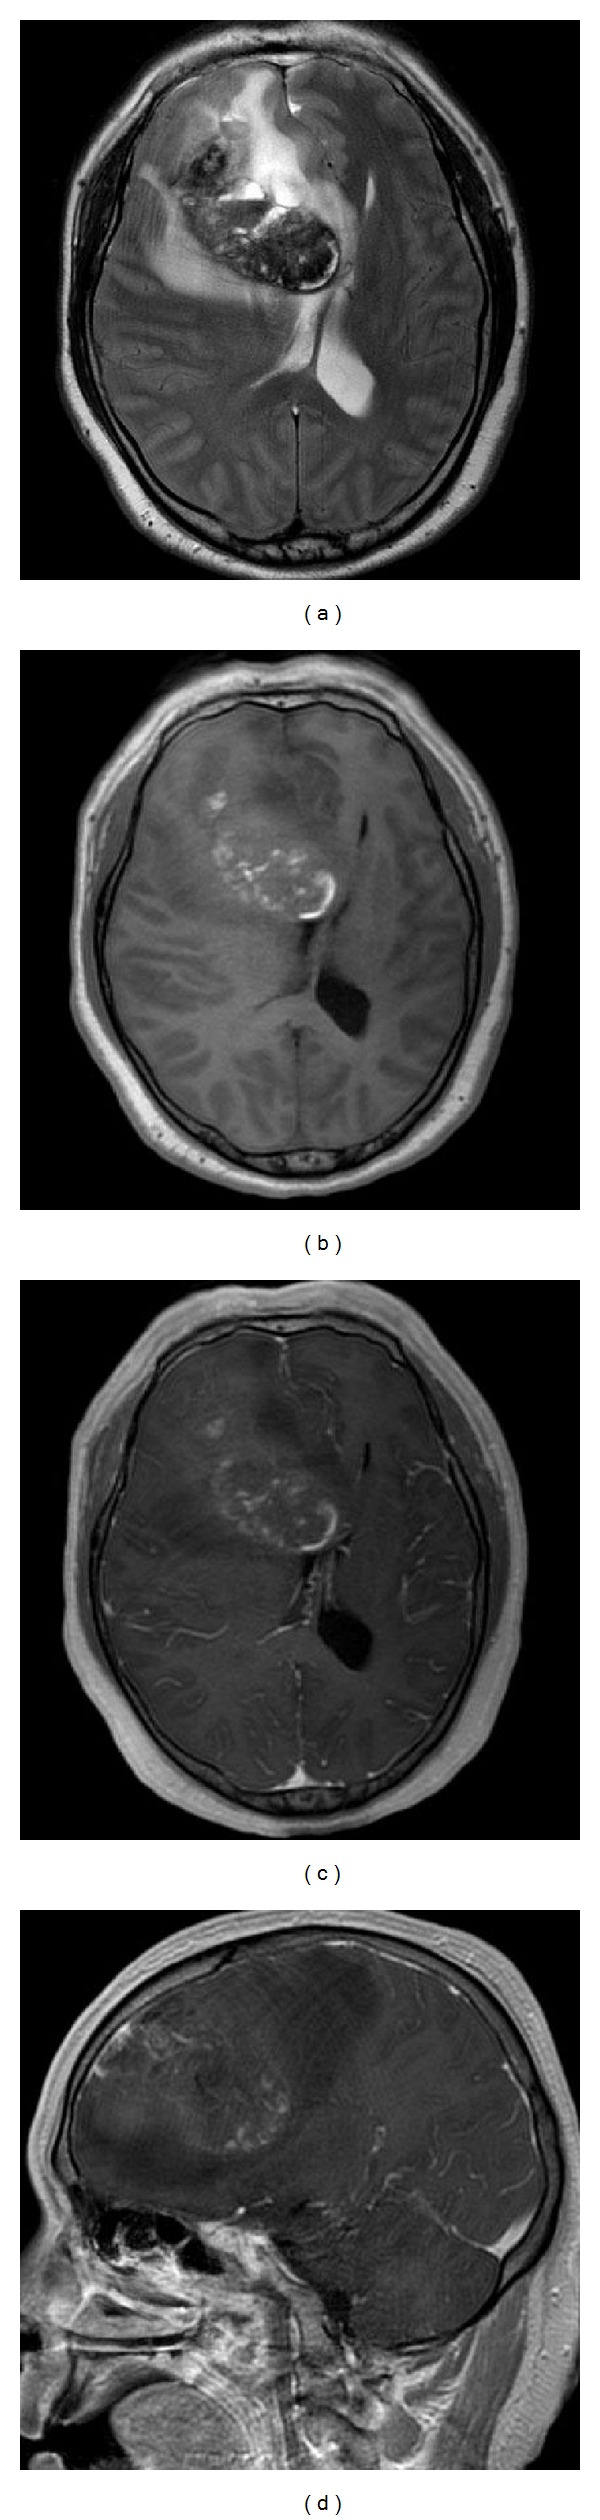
Axial T2-weighted imaging (WI) (a), axial T1-WI (b), axial T1-WI with gadolinium (c), and sagittal T1-WI with gadolinium (d) magnetic resonance images showing a huge tumor with intratumoral hemorrhage in the right frontal lobe that led to subfalcial and transtentorial herniation.
